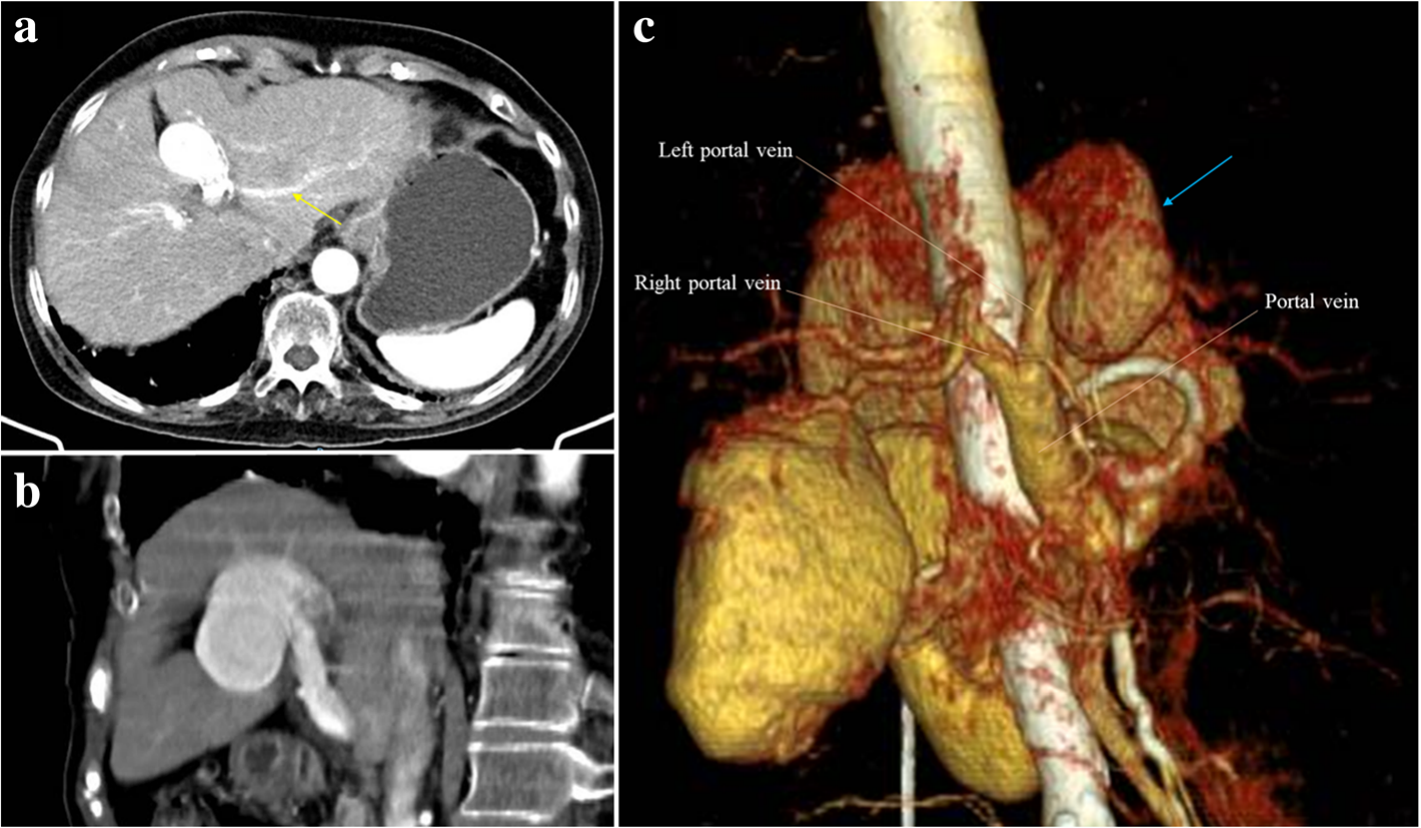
Aneurysm of the PVS. a, b Axial and oblique MPR images from late arterial phase CT shows an aneurysmal dilatation of left PV with about 3 cm of greater axis. Note the left PV branches arising directly from the aneurysm (yellow arrow). c VRT from late arterial phase best depict the origin of the aneurysm in the PVS
